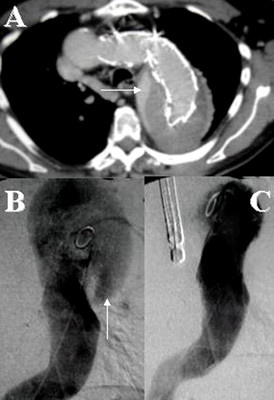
Follow-up CT-A showed late type Ib endoleak (*arrow*) after SG treatment of a type B dissecting aneurysm (A). Preliminary DSA (B) confirmed the re-perfusion of the false channel through a distal re-entry tear (*arrow*): additional SG was used to seal the aneurysm as confirmed by final DSA (C) with TEE control.
